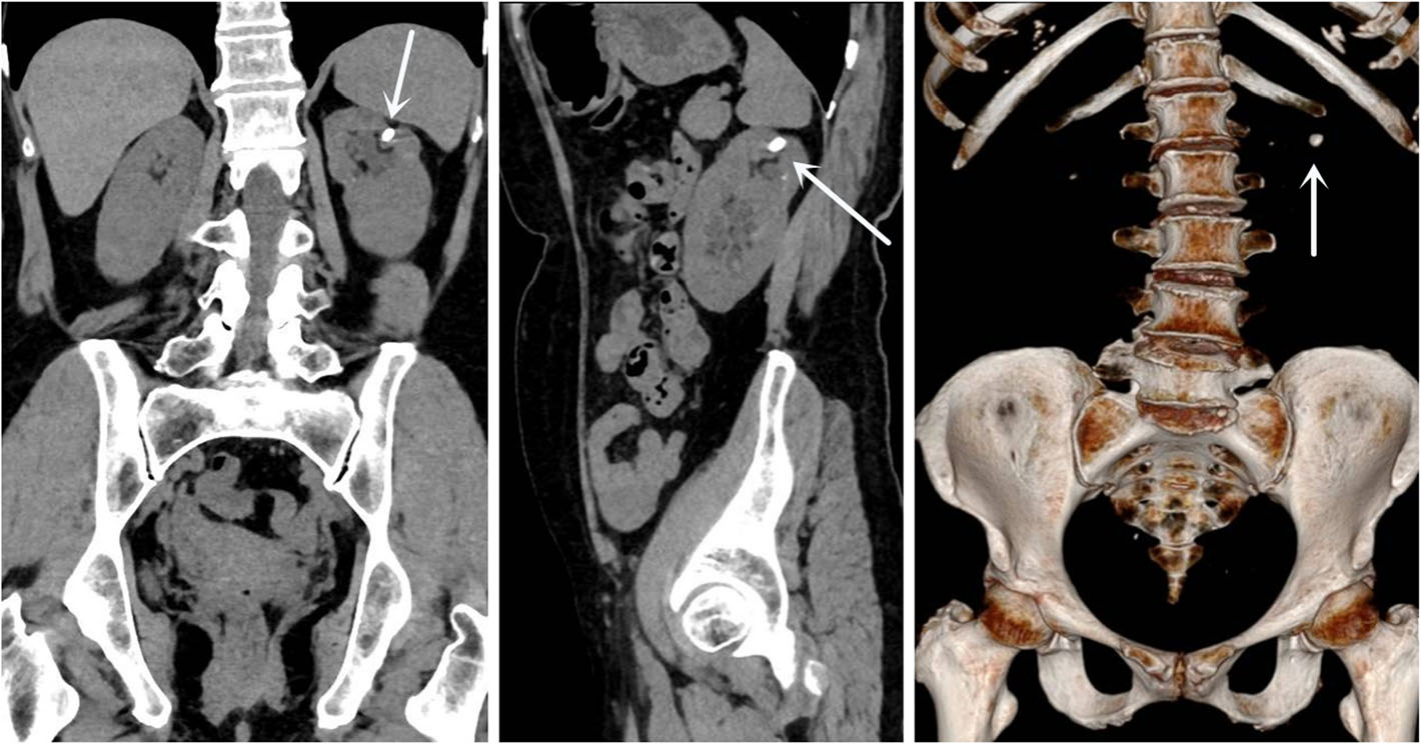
The stone of calyceal diverticulum was located in the upper kidney of left incomplete duplex kidneys by CT scan
